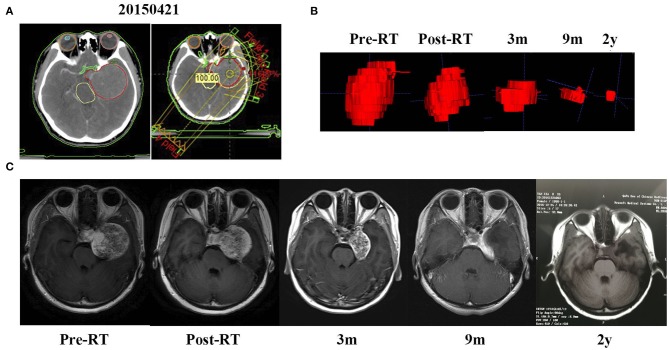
A mass occupying the left cavernous sinus of a 24-year-old woman (patient 1) was shrunk gradually after RT. (A) Treatment plan based on CT scan. (B) The 3D imaging of the mass before RT and at a different time of follow up after RT. (C) MRI scan before RT, the day after RT completion and 3 months, 9 months, and 2 years after RT.
